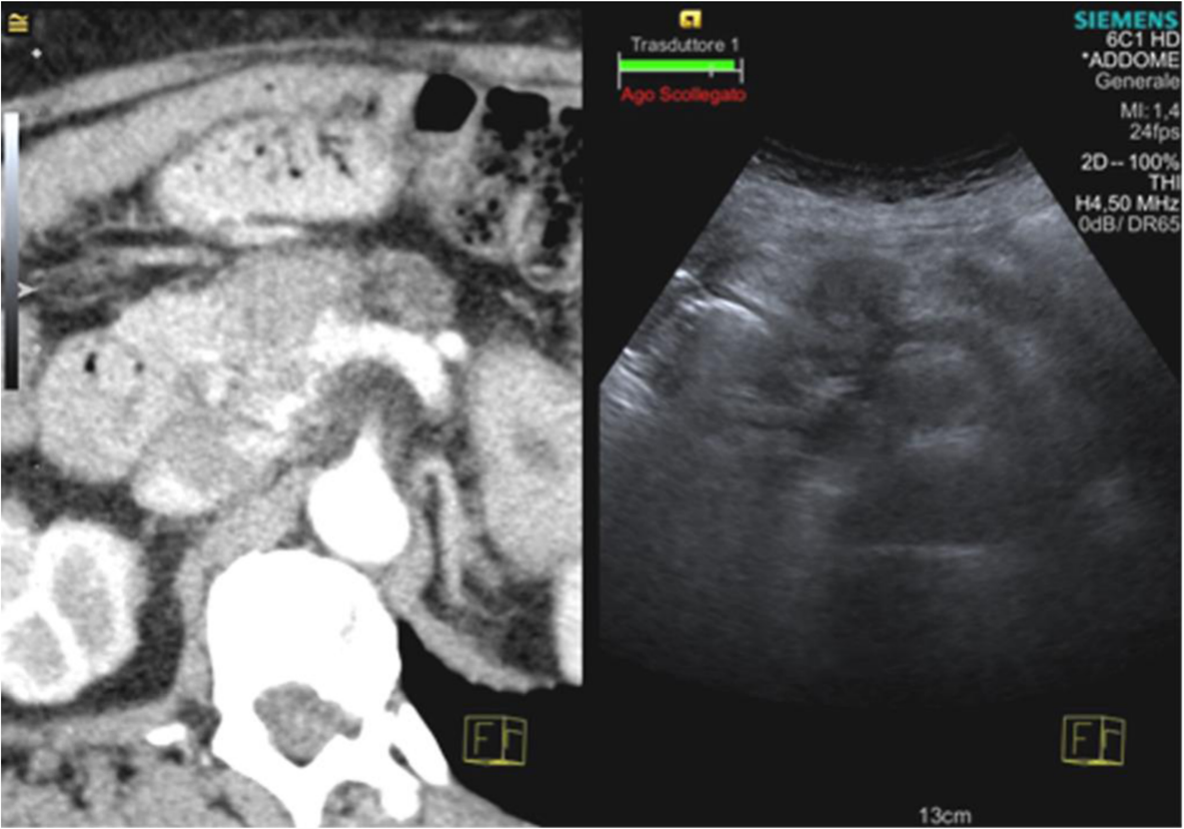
CT-US fusion imaging. Biopsy of pancreatic neck carcinoma, hypodense on CT and hypoechoic on the US
